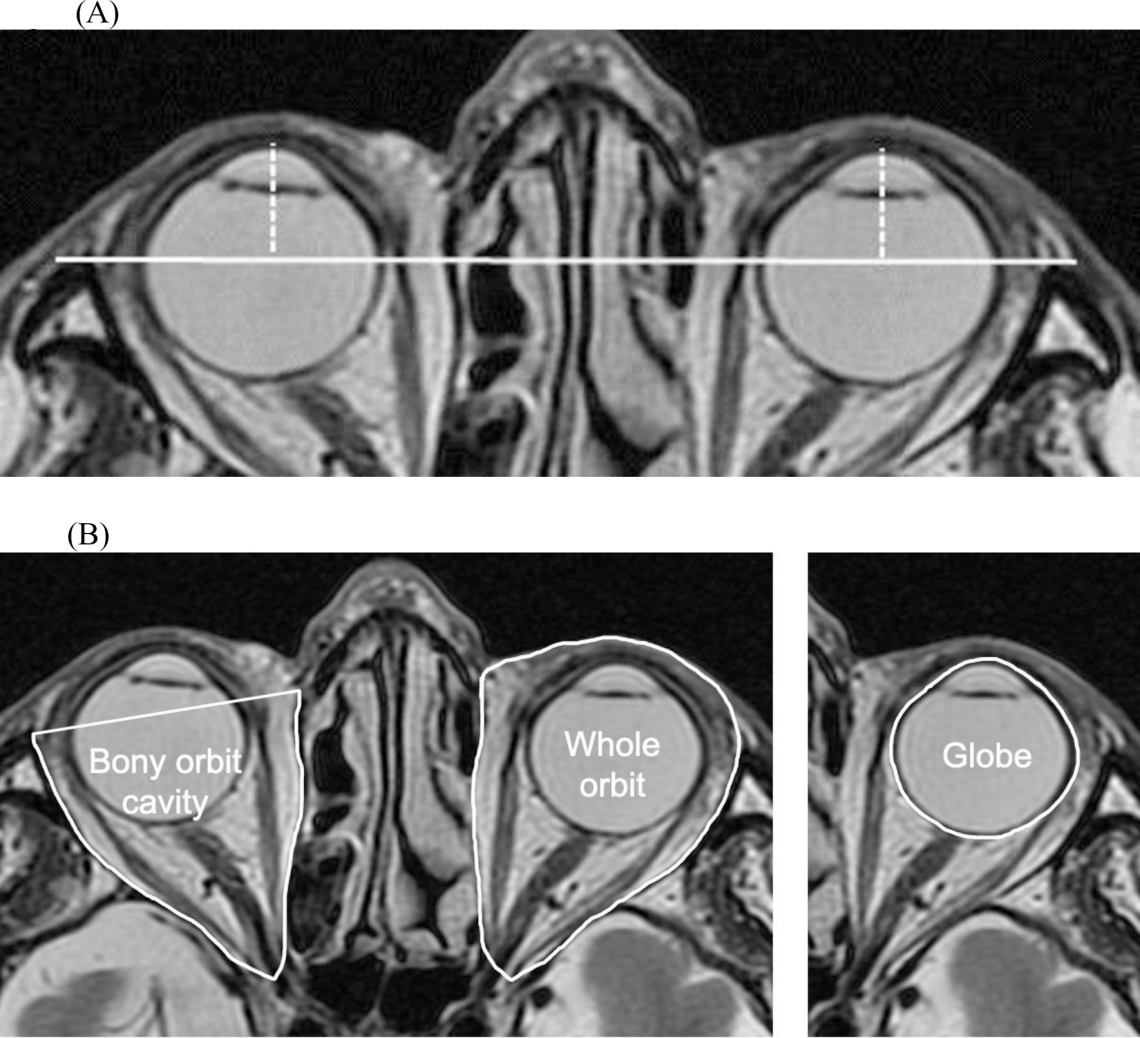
(A) Measurement of the proptosis by axial magnetic resonance imaging. A line was drawn between the bilateral frontal processes of the zygomatic bones (solid line). The proptosis value was measured as the perpendicular distance from the top of the cornea to the line (dotted line). (B) Measurement of the cross-sectional areas of the orbital tissues on T2-weighted images. Axial slice showing the cross-sectional areas of the bony orbital cavity in the right orbit and that of the globe and the whole orbit in the left orbit.
